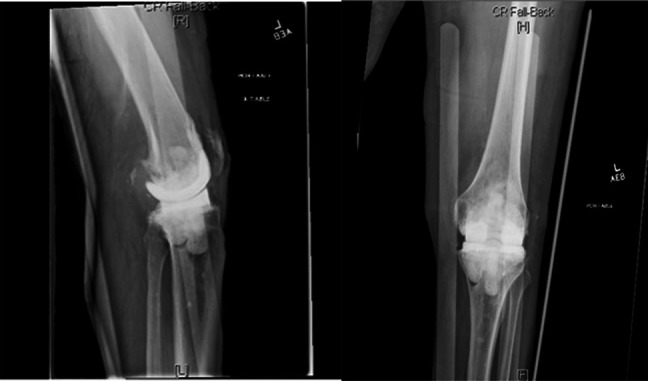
A, B, Postoperative radiographs with placement of the antibiotic spacer exemplifying proper anatomic alignment and no evidence for acute complication.
